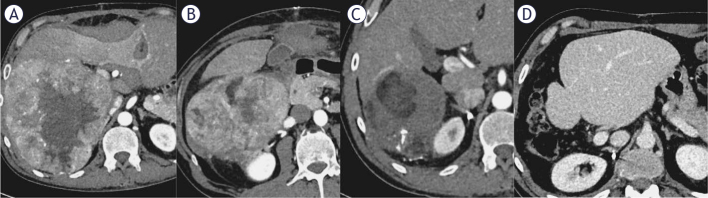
50-year-old male with 170 mm hepatocellular carcinoma (HCC), portal vein tumor thrombosis (Japanese classification Vp4), inferior vena cava (IVC) tumor thrombosis (Japanese classification Vv3) and Child A cirrhosis (A). Follow-up CT scan showed a partial response 2 months after transarterial radioembolization (TARE) (B), followed by 7 cycles of atezolizumab/bevacizumab, resulting in a complete response after 4 months (C). A right hemihepatectomy with marginal excision of the vena cava was performed, with no disease progression upon follow up (D).
